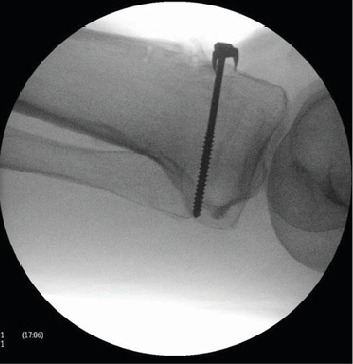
Intraoperative lateral radiograph showing fixation with spiked washer and partially threaded cancellous screws.
